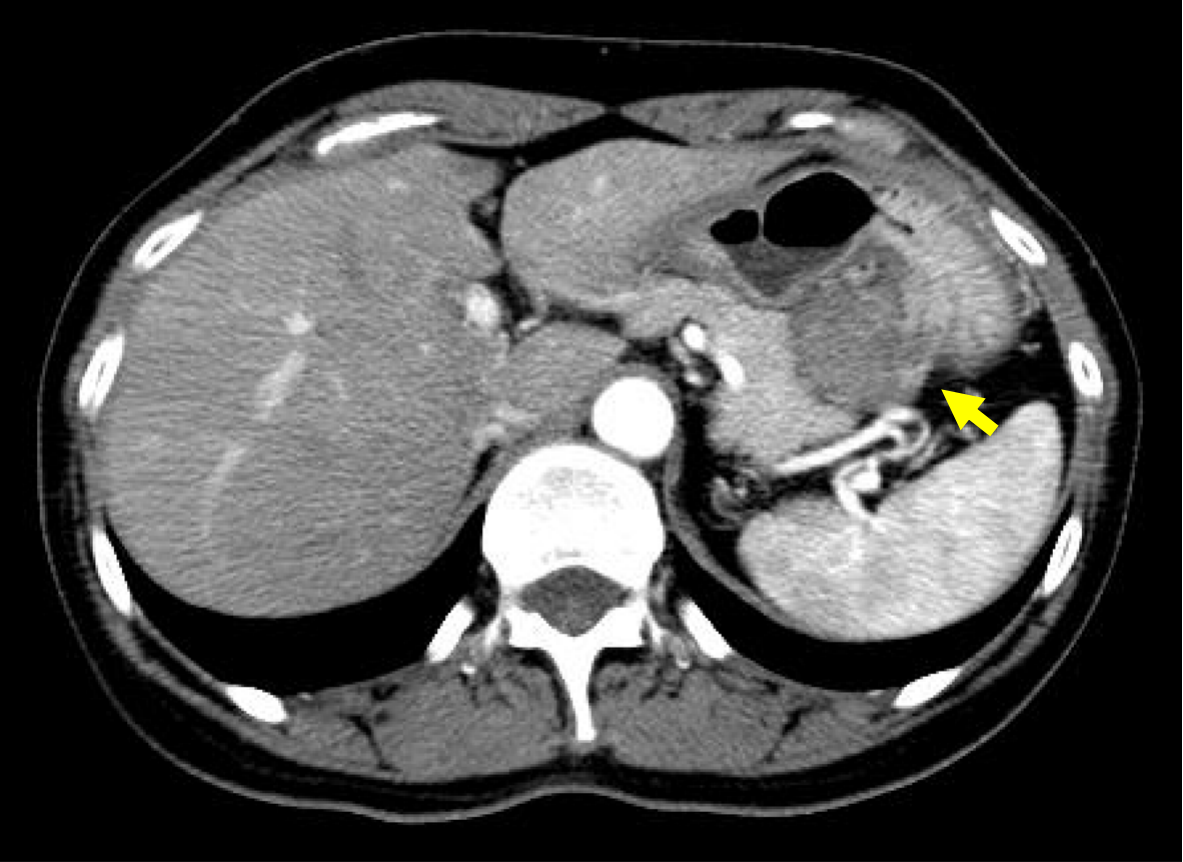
Dynamic and contrast-enhanced CT showed a 30 × 25 × 50-mm solid mass (yellow arrow) between the stomach and the pancreas tail. The lesion had a smooth margin and an inhomogeneously moderate enhancement in the arterial phase
